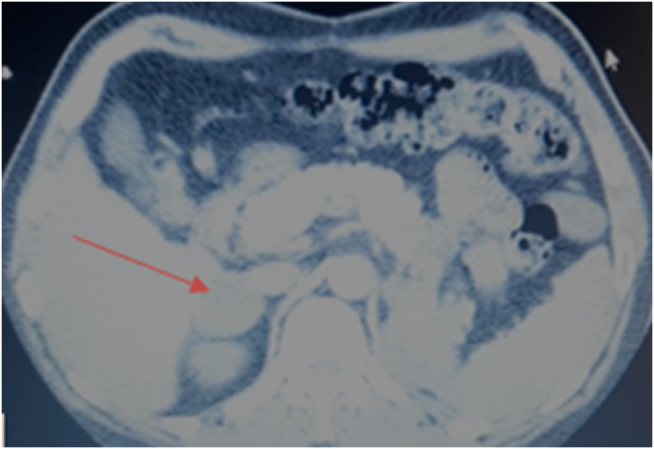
Contrast-enhanced CT scan of the abdomen showing an enhanced right adrenal mass (arrow).
